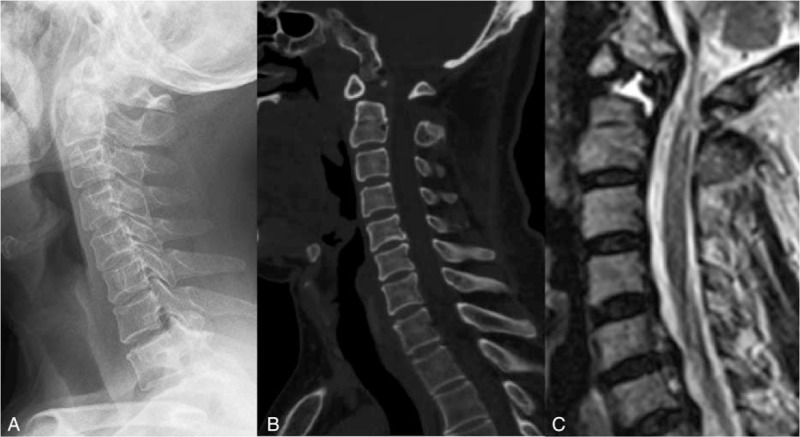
The atlantoaxial instability resulted in mild atlantoaxial dislocation, as observed on the preoperative X-ray (A) and CT scan (B). (C) MRI showed that the spinal cord was compressed by the posterior atlas arch. CT = computed tomography, MRI = magnetic resonance imaging.
